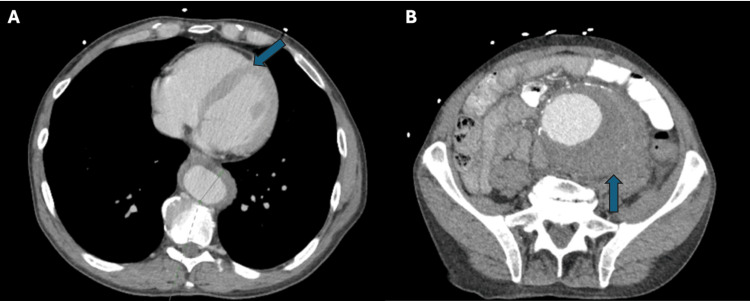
CT scan of the chest and abdomen Chest (A) and abdominal (B) CT scans demonstrate a maximum 3.5 cm aneurysmal dilation of the descending thoracic aorta and an 8.9 x 7.8 cm fusiform infrarenal aneurysm with hemorrhage on the left aspect, respectively.
